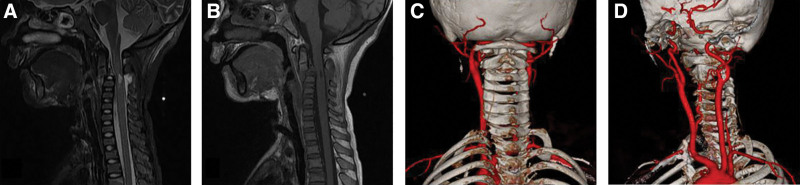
Preoperative cervical MRI (A and B) showed C2–C3 level spinal cord injury, C2 lower margin fracture, C2 IV° anterior slippage, and C2–C3 space widening. CTA enhancement of the cervical vessels (C and D) showed that the left vertebral artery V1 and 2 segments were occluded. The communication branch between the left external carotid artery and the left vertebral artery was developed in the C2 plane, and there was no vertebral artery riding.
